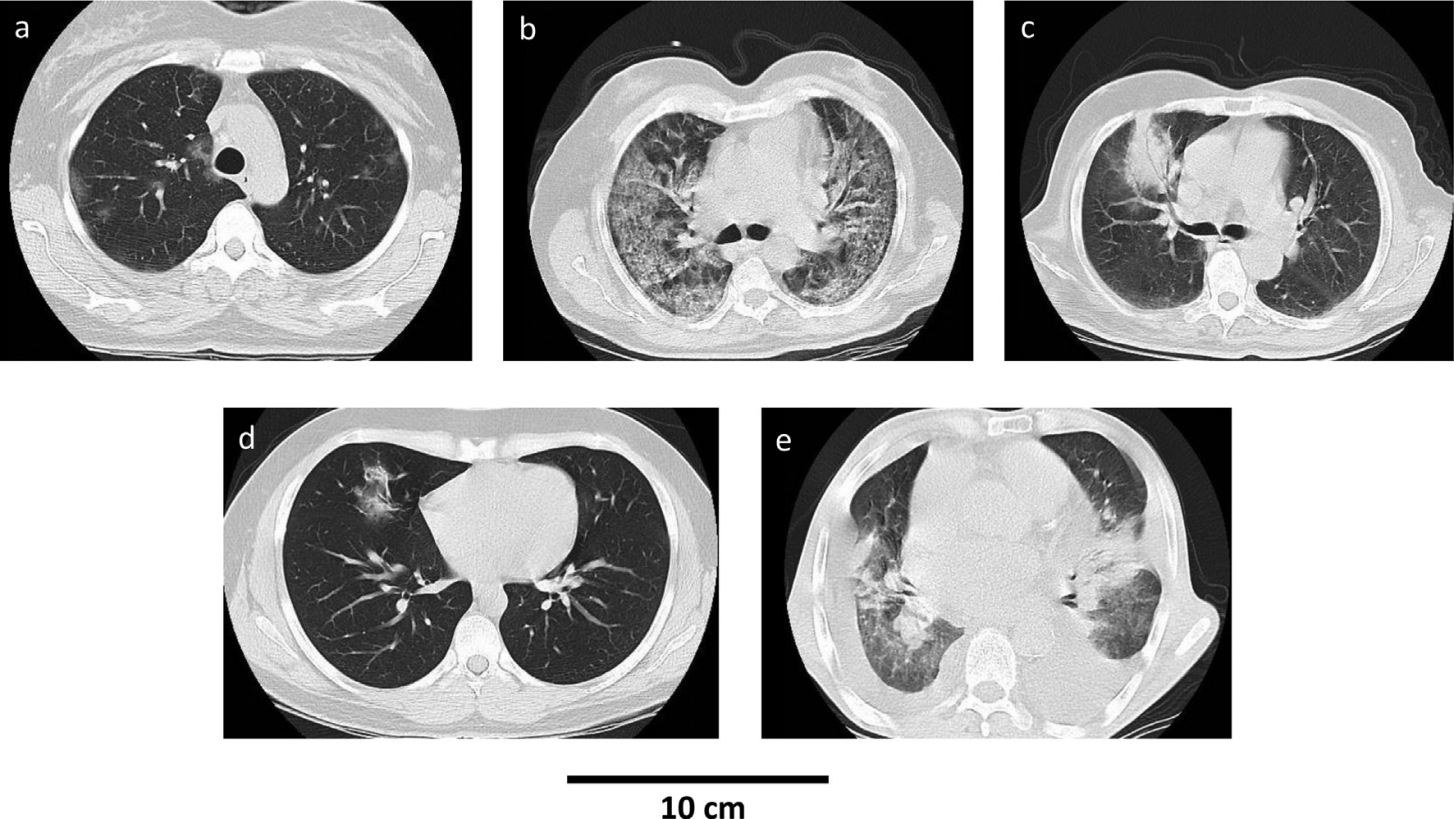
Transverse CT scans a) Low dose lung HRCT shows multiple patchy ground glass opacities in both lungs as sign of COVID-19 pneumonia. b) Low dose lung HRCT shows cardiomegaly and diffuse crazy paving appearance in lungs suggesting COVID-19 pneumonia. c) Low dose lung HRCT shows cardiomegaly and air space consolidation in RML, suggesting lobar pneumonia. d) Low dose lung HCRT shows a consolidation patch in RML with surrounding ground glass opacities (halo sign). e) Low dose lung HRCT showed cardiomegaly, bilateral peribronchiovascular consolidation, pleural effusion, DDX was pulmonary edema and Covid-19 pneumonia. Note for all of cases the results of RT-PCR test are positive.
